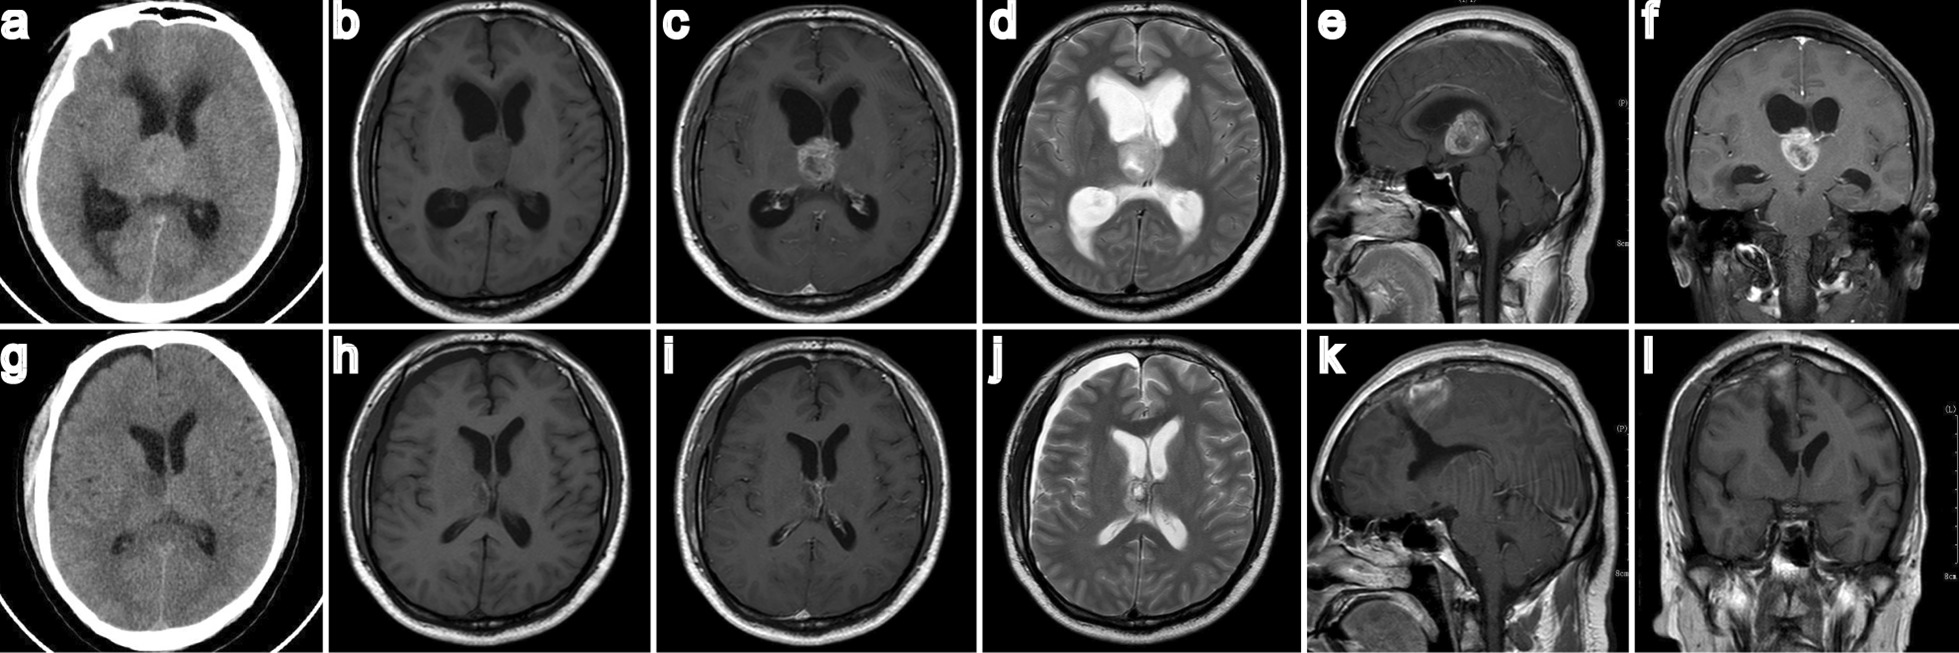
Case 1. Cranial CT and MRI revealed a unilateral thalamus located in the right thalamus. Preoperative CT and MRI (a–f). Postoperative CT and MRI confirmed total resection (g–l)
